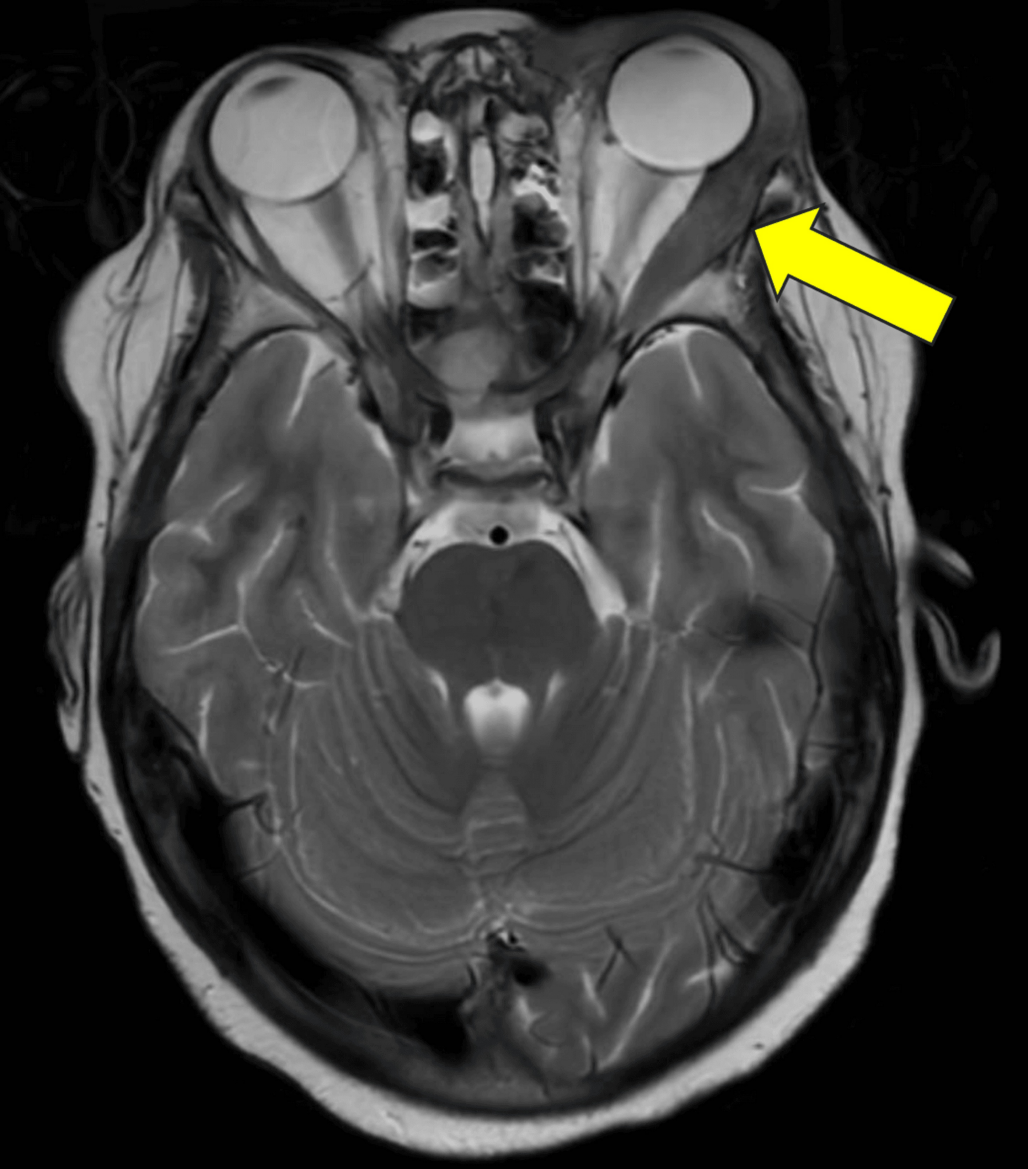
Axial T2-weighted MRI demonstrating a lesion involving the lateral rectus muscle and orbit of the left eye (yellow arrow).
